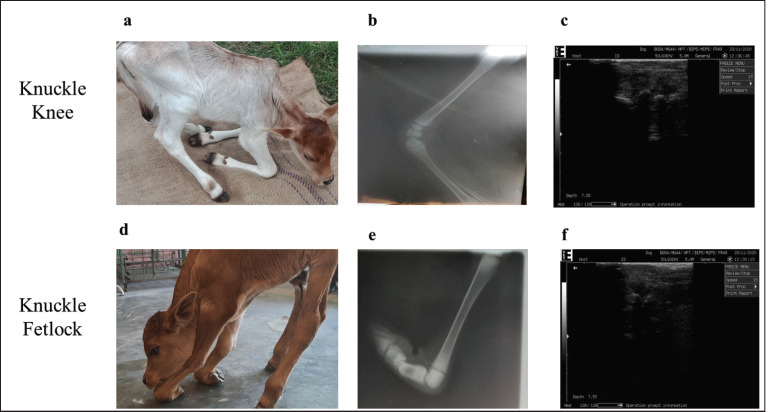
Preoperative clinical, radiographic and ultrasonographic examination in calves a) clinical appearance, b) radiographic examination and c) ultrasonographic examination in knuckle knee. Similarly, d) clinical appearance, e) radiographic examination and f) ultrasonographic examination of calves with knuckling of the fetlock.
